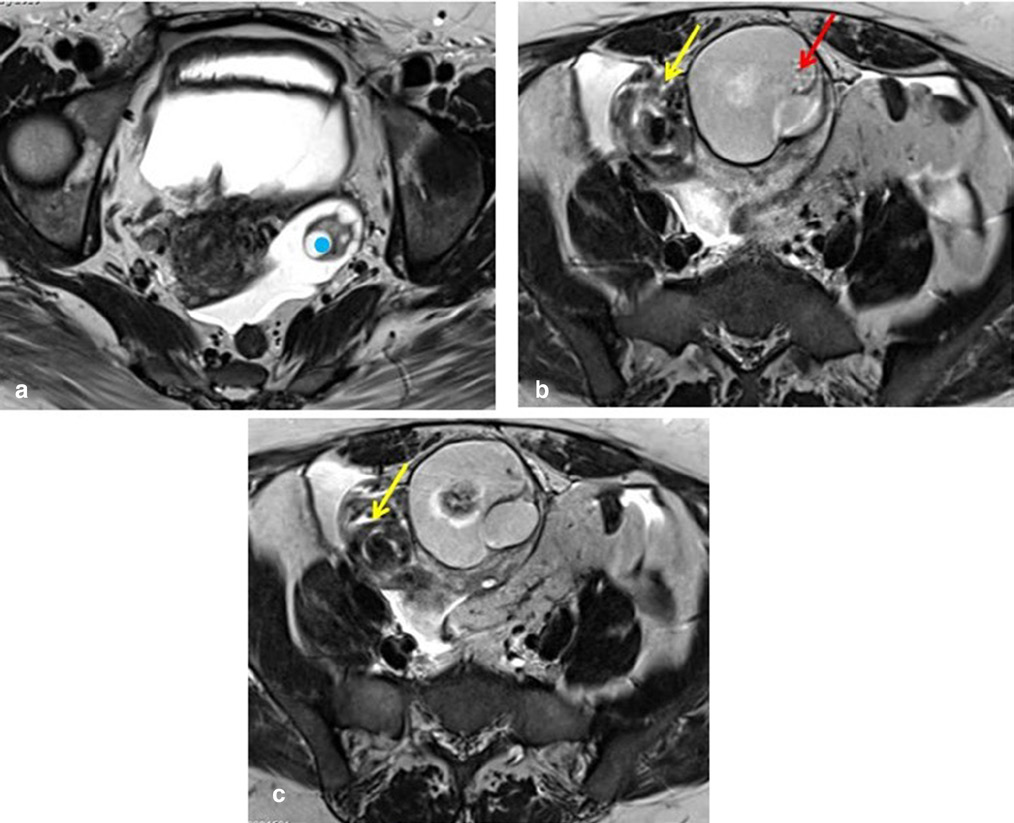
(A, B, C): Axial T2 MRI. Figure A shows the normal left ovary
